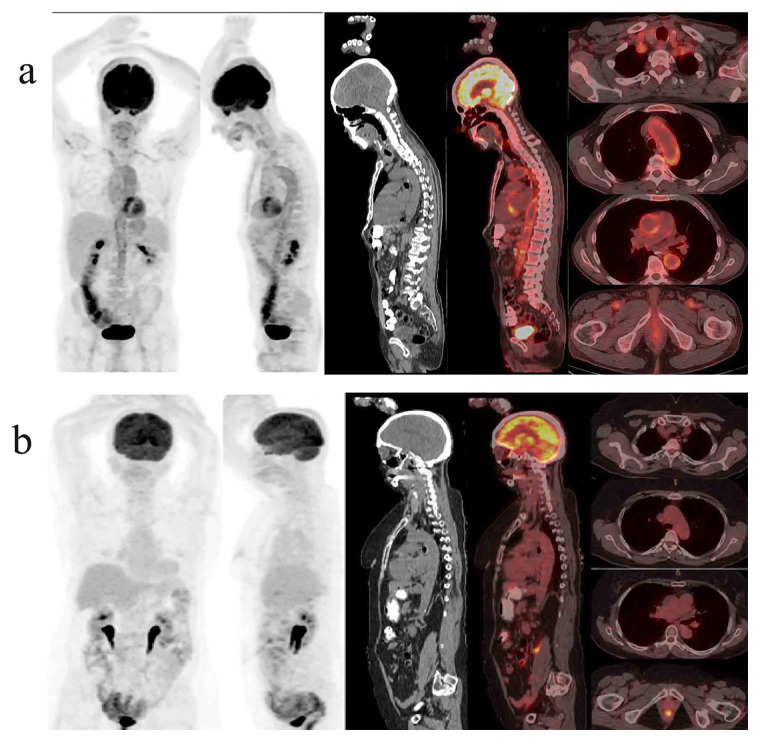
Representative PET/CT images showing maximum (PETVAS = 27) (a) and minimum (PETVAS = 0) (b) scores.
